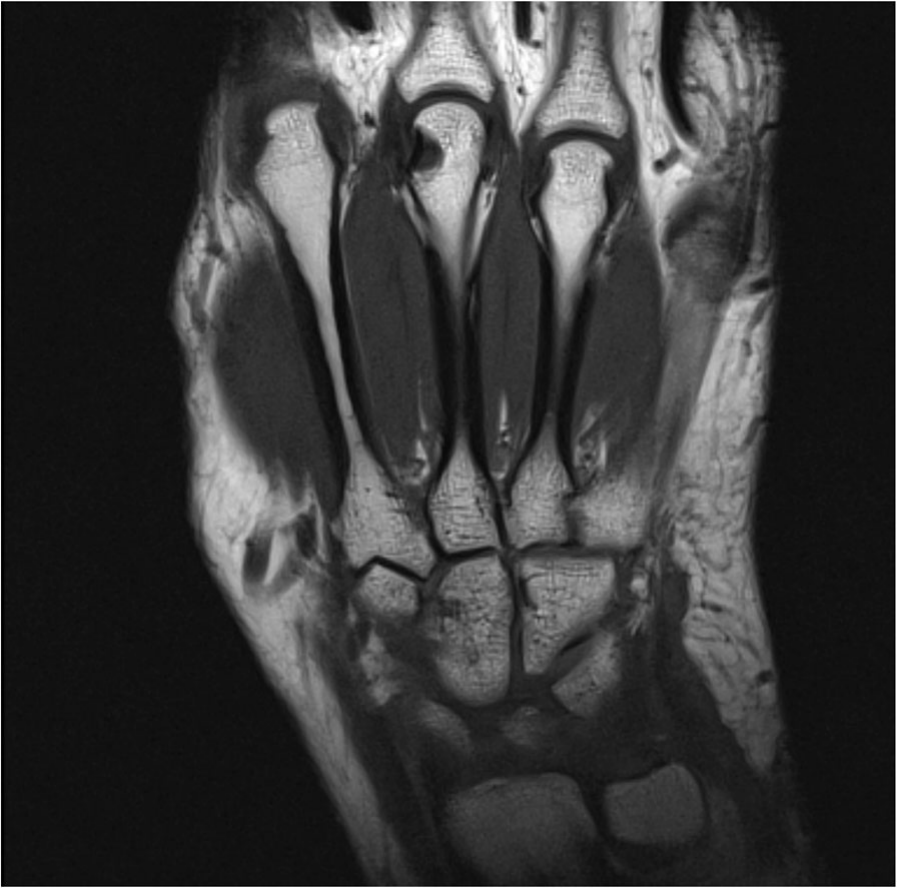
Example of a patient’s hand with erosion in the metacarpophalangeal III joint (coronal T1-weighted turbo spin echo)
